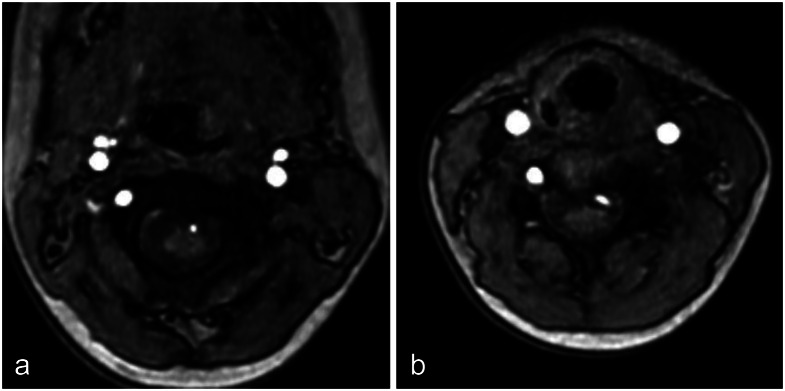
Axial source images of cervical MRA using a 3 T magnetic resonance imaging scanner. a: Axial image at the level of the C2 vertebra shows absence of the left transverse foramen. b: Axial image at the level of the C4 vertebra also shows absence of the left transverse foramen. These findings support a congenital defect of the left vertebral artery pathway
